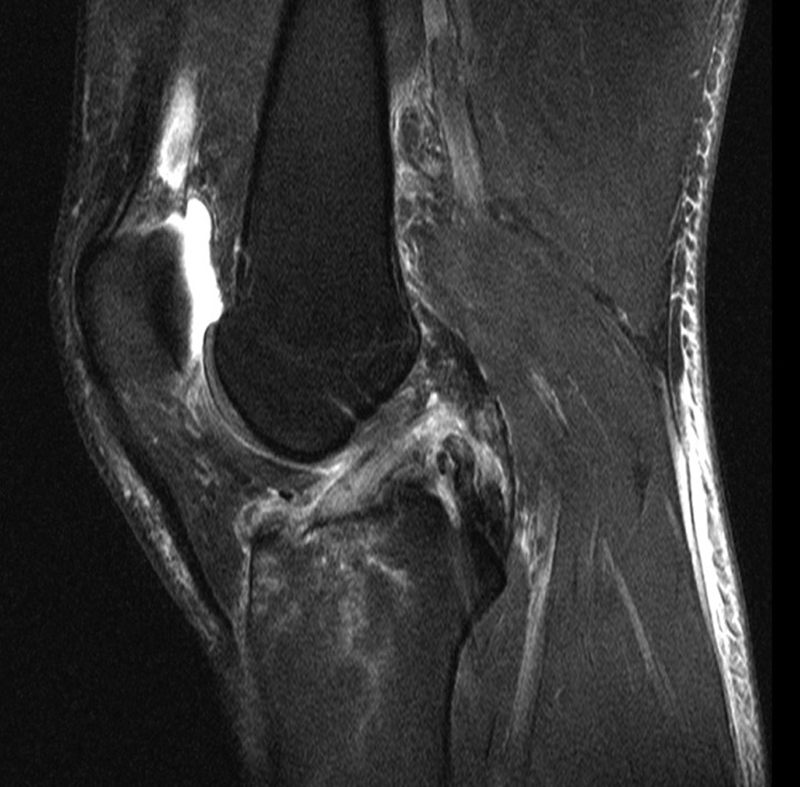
Magnetic resonance imaging of the injured knee showing the partial tear of the anterior cruciate ligament and the complete rupture of the posterior cruciate ligament.
